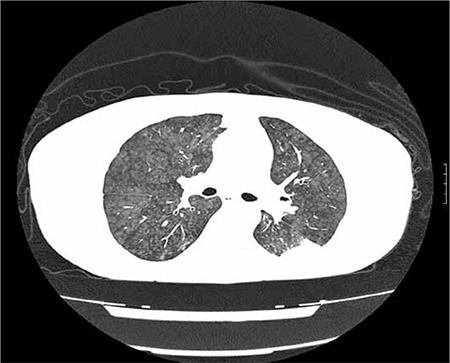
HRCT at the level of the upper lobes shows nodular ground glass opacities with a predominately centrilobular distribution.
